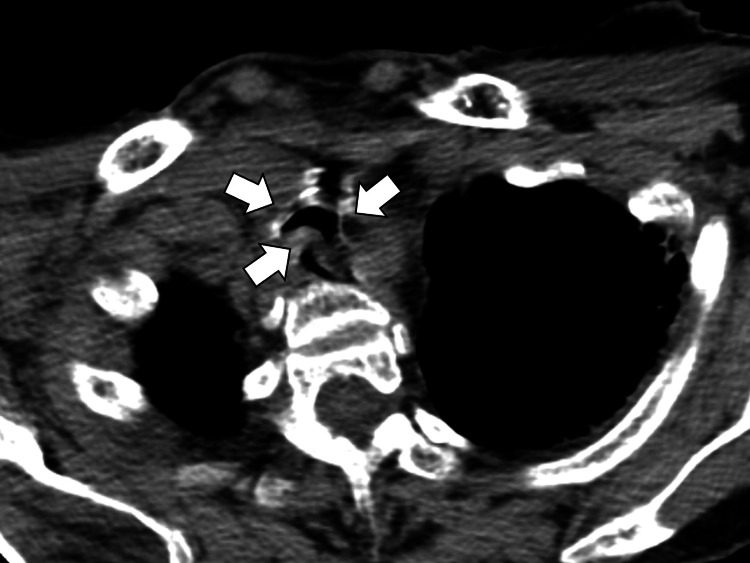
Chest computed tomography demonstrating the elevation of the membranous portion of the main bronchi during inspiration, which suggested tracheomalacia (white arrows)
